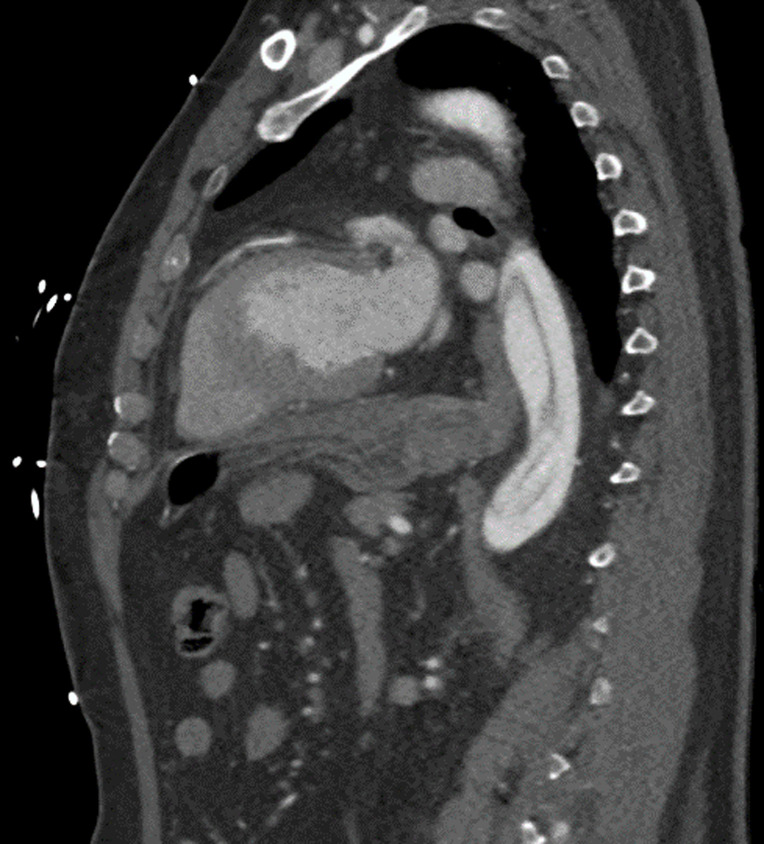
intimal flap on chest computed tomography angiogram
